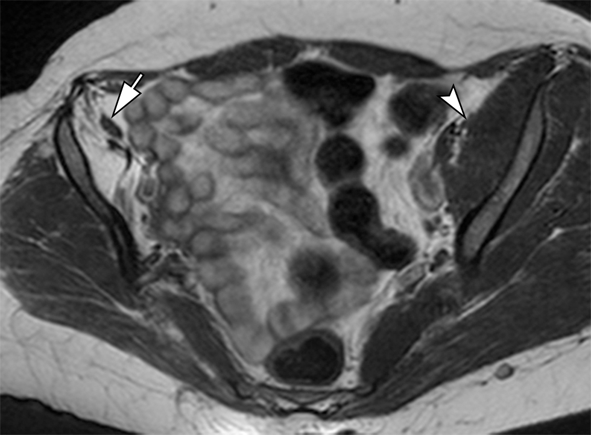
Femoral nerve lesion. A 44 year-old male with a right femoral nerve iatrogenic injury related to a hip prosthesis surgery. Axial T1-weighted image shows thickening of the right femoral nerve (arrow) and complete atrophy of the iliopsoas muscles. The left femoral nerve is normal (arrowhead)
